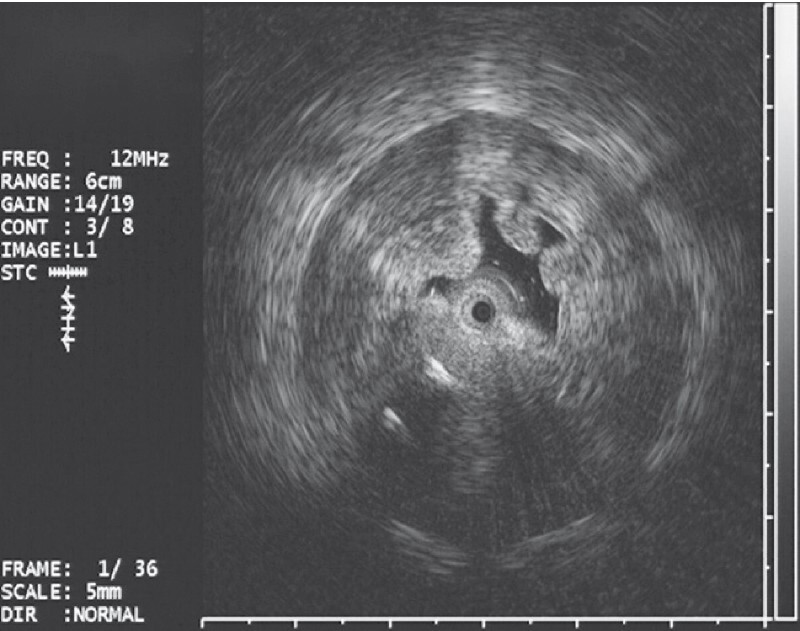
Endoscopic ultrasonography images of a gastric antrum submucosal lesion.
